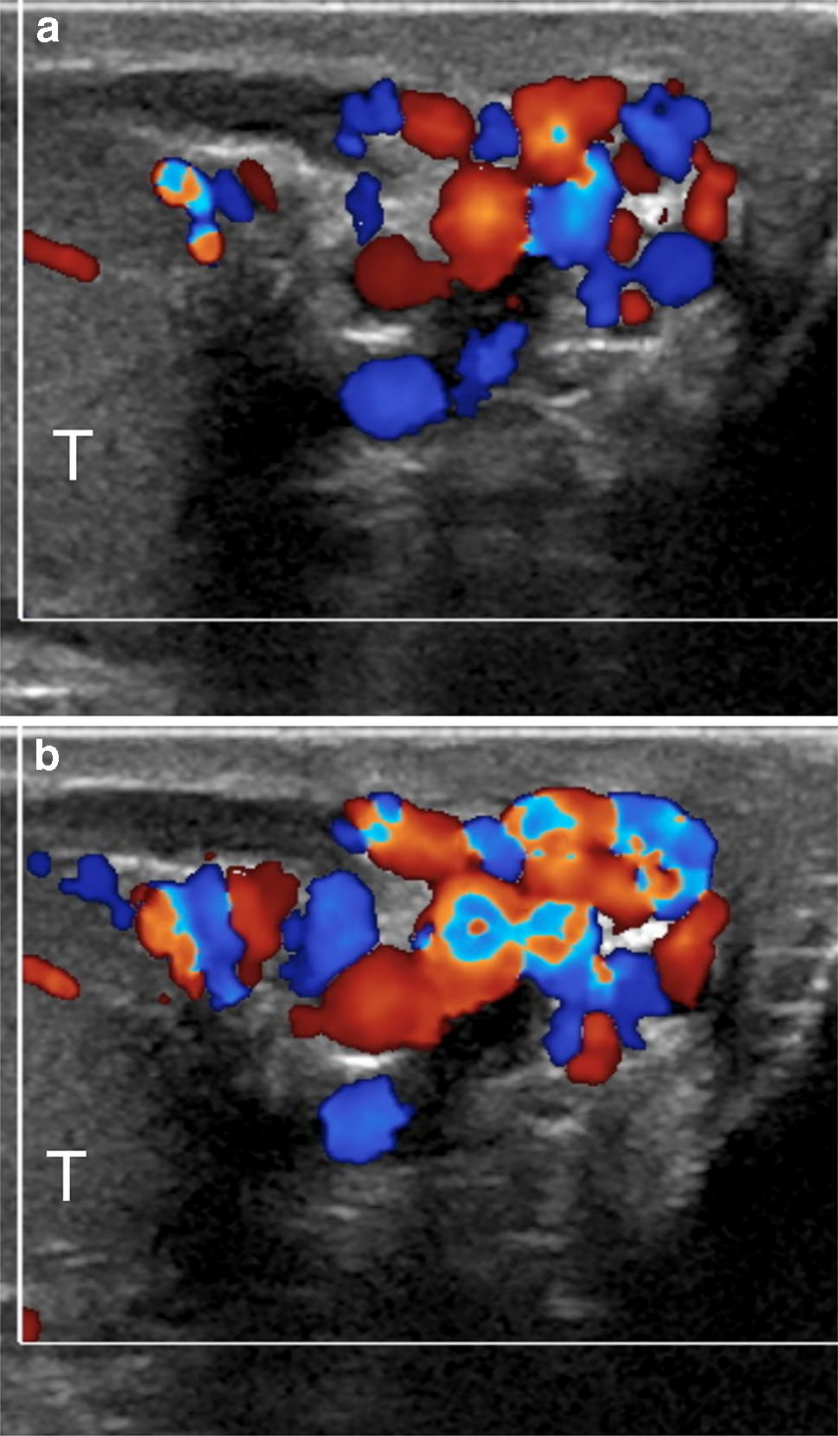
Sarteschi’s grade IV varicocele. Colour Doppler images obtained in supine position at rest (a) and while standing during Valsalva (b). Dilated veins with reflux are visible also at rest. Reflux increases while standing during Valsalva (T = testis)
